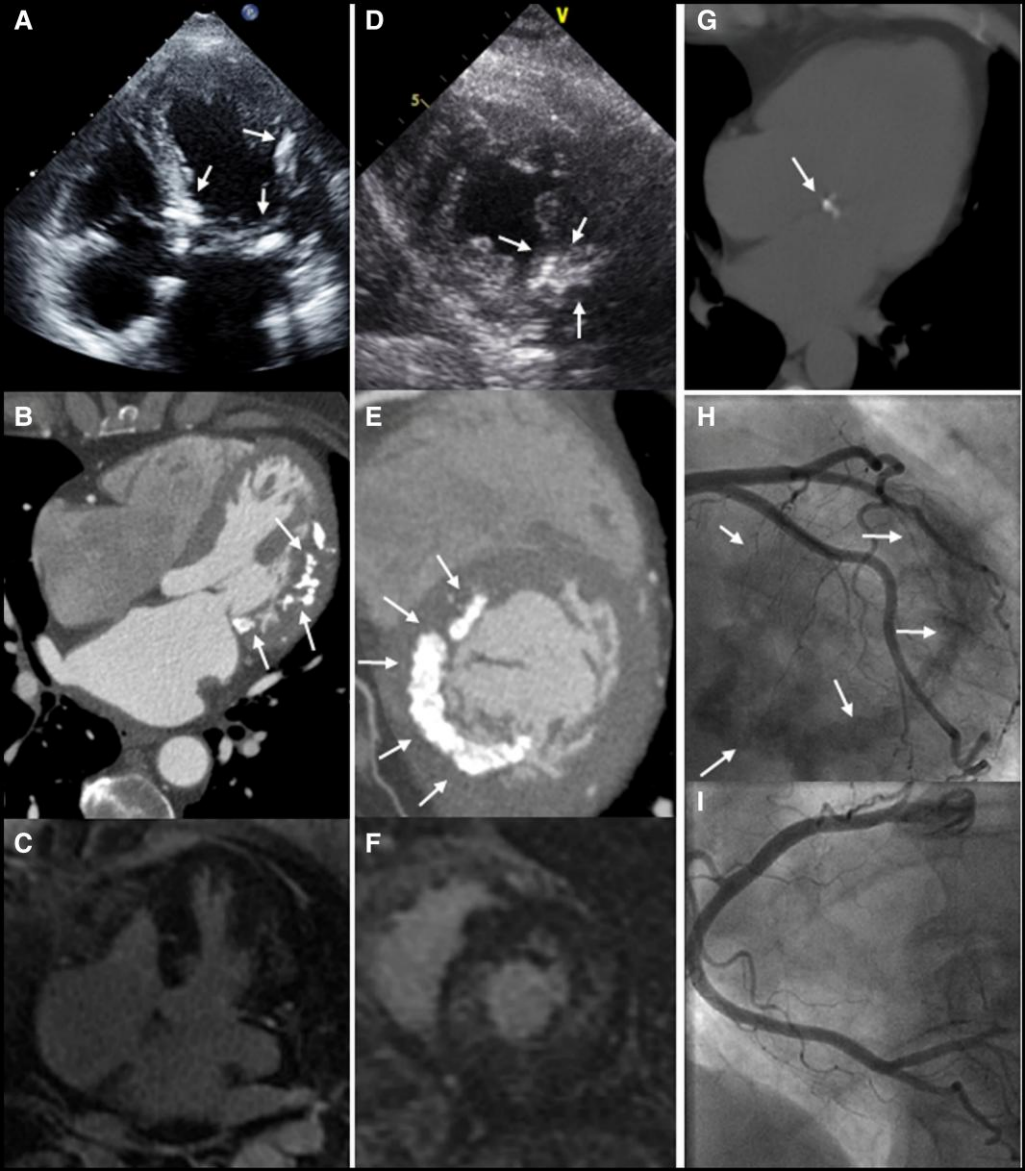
Different imaging modalities demonstrating extensive myocardial calcifications. Four-chamber view of the (*A*) echocardiographic, (*B*) cardiac computed tomographic angiography, and (*C*) cardiac magnetic resonance imaging studies of Patient 1 demonstrating gross mitral annular and myocardial calcifications (arrows) along with diffuse, patchy, mid-myocardial delayed enhancement involving the lateral wall. The corresponding short-axis views of the (*D*) echocardiographic, (*E*) cardiac computed tomographic angiography, and (*F*) cardiac magnetic resonance imaging studies also demonstrate gross myocardial calcifications (arrows) along with diffuse, patchy, mid-myocardial delayed enhancement involving the anterolateral wall. (*G*) Chest computed tomography obtained from the same patient 9 years prior to the present presentation which shows only minimal mitral annular calcification with no myocardial involvement. (*H*, *I*) Coronary angiography demonstrating evidence of severe myocardial calcifications (arrows) and no evidence of coronary artery disease within the (*H*) left and (*I*) right coronary arteries along with.
